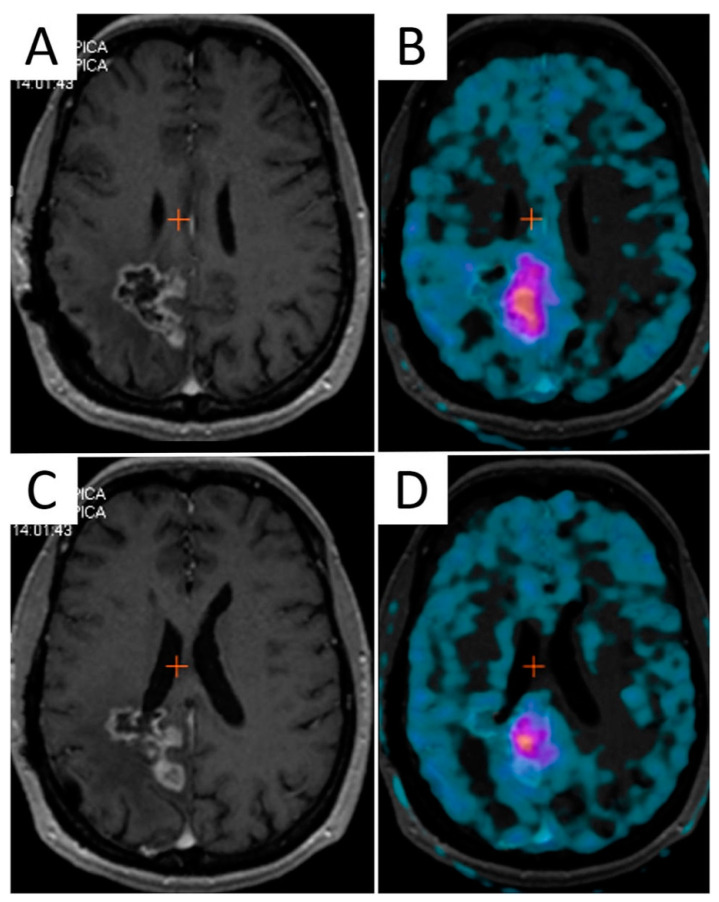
The mismatch between contrast-enhanced T1-weighted MRI and 3,4-dihydroxy-6-[^18^F]fluoro-L-phenylalanine (F-DOPA) PET/CT in a 45-year-old female patient with recurrent GBM after multimodal first-line therapy. Two axial contrast-enhanced T1-weighted images (A,C) along with corresponding F-DOPA PET/CT slices (B,D) identifying different volumes of disease.
